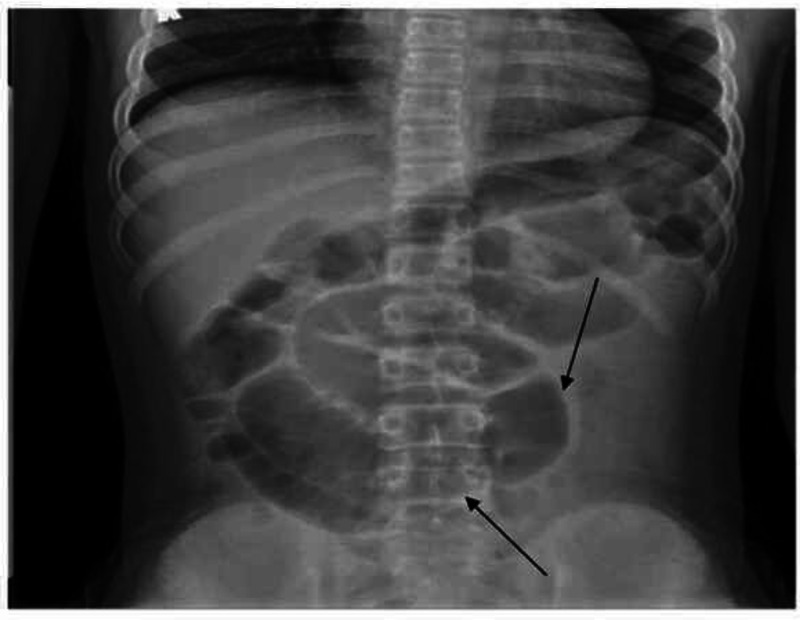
X-ray abdomen flat The image shows an abnormal bowel gas pattern with multiple abnormally dilated and air-filled loops of SB (black arrows). Partial distal SBO cannot be excluded although alternatively there may be an ileus SBO: small bowel obstruction
